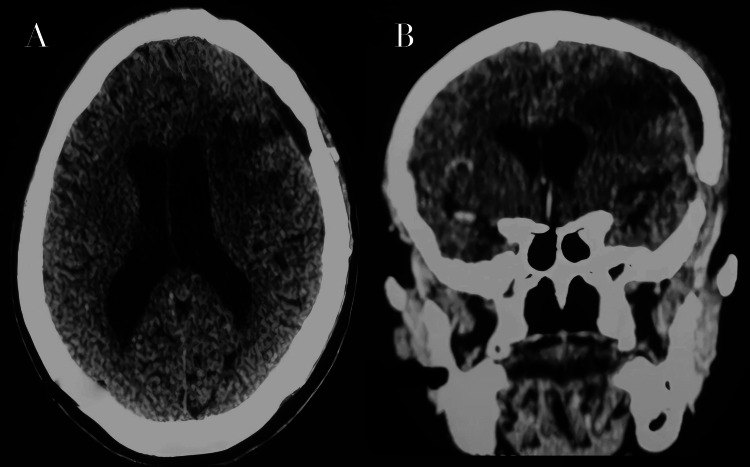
Follow-up contrast-enhanced CT scan performed one month after surgery. Axial (A) and coronal (B) views show postoperative changes without evidence of residual enhancing tumor. CT, computed tomography
